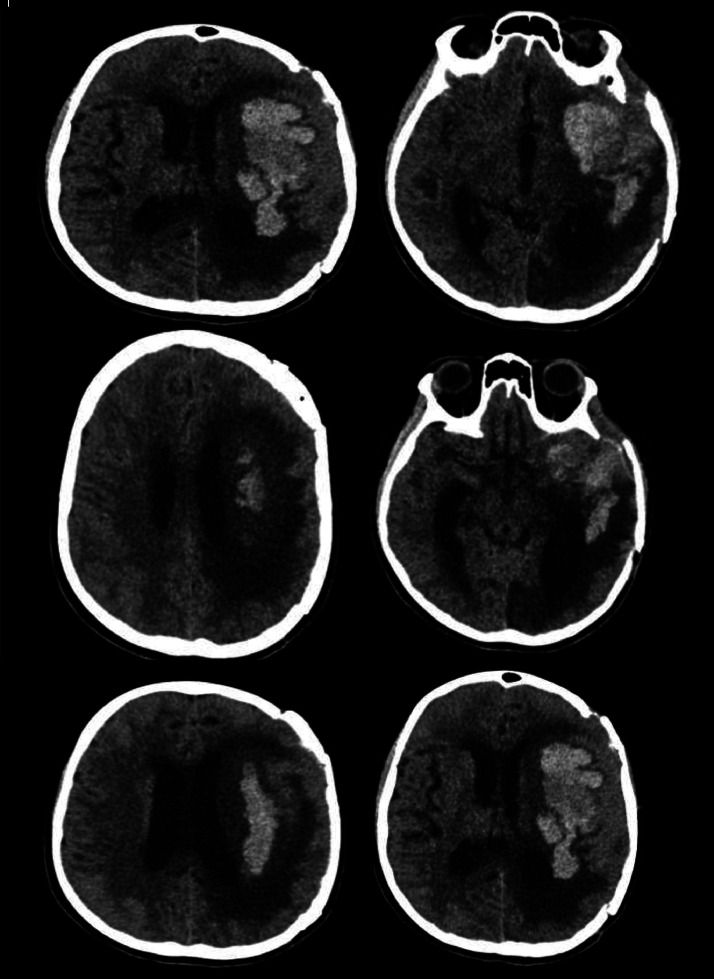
Non-contrast axial CT scan postcraniostomy for the third time. The non-contrast axial CT scan of the brain reveals a large, heterogeneous, hyperdense hemorrhage in the left parietal lobe, with surrounding hypodense vasogenic edema, exerting significant mass effect and causing a midline shift with ventricular compression. The findings are highly suggestive of an acute intracerebral hemorrhage. Possible intraventricular extension is noted, raising concern for hydrocephalus.
